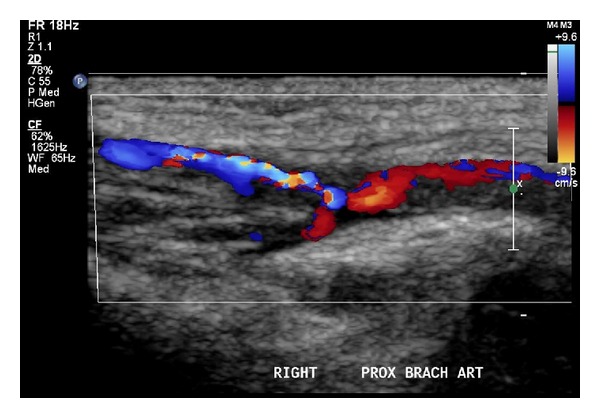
Ultrasound scan of the right proximal brachial artery demonstrating a substantial non-occlusive hypo-echoic thrombus.
